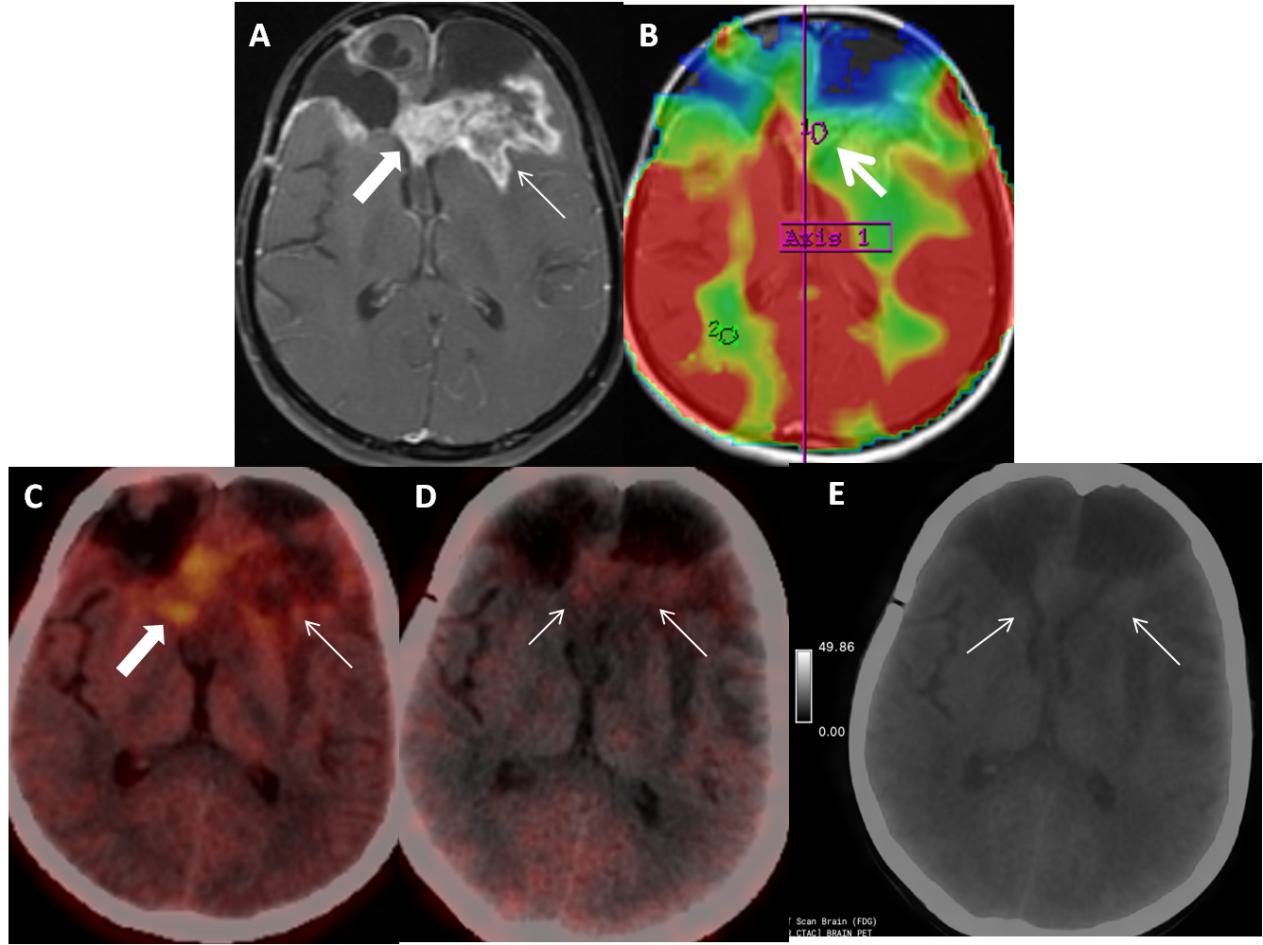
Patient 3 is a 39-year-old female, treated case of IDH-mutant WHO Grade IV glioma (last treatment in June 2019), recurrence on surveillance MRI (September 2021), underwent re-surgery, and received adjuvant temozolamide. Patient presented with seizures in December 2021. Axial post contrast T1-weighted image (A, arrow) shows predominantly feathery enhancement (arrow) with few areas of thick peripheral enhancement suggestive of Swiss cheese appearance (block arrow) and hypoperfusion (B, arrow). Correlative axial FET PET/CT image show tracer uptake (C, arrow) with T-W ratio of 2.5 suggestive of post treatment change. Axial FAPI PET/CT and gray scale FAPI PET image (D, E; arrows) shows low intensity diffuse tracer uptake in the corresponding region equal to background uptake interpreted as negative FAPI PET. Patient was asked to follow up with short interval MRI, which was unchanged thus confirming the diagnosis of post-treatment change. FET: fluoro-ethyl tyrosine; MRI: magnetic resonance imaging
